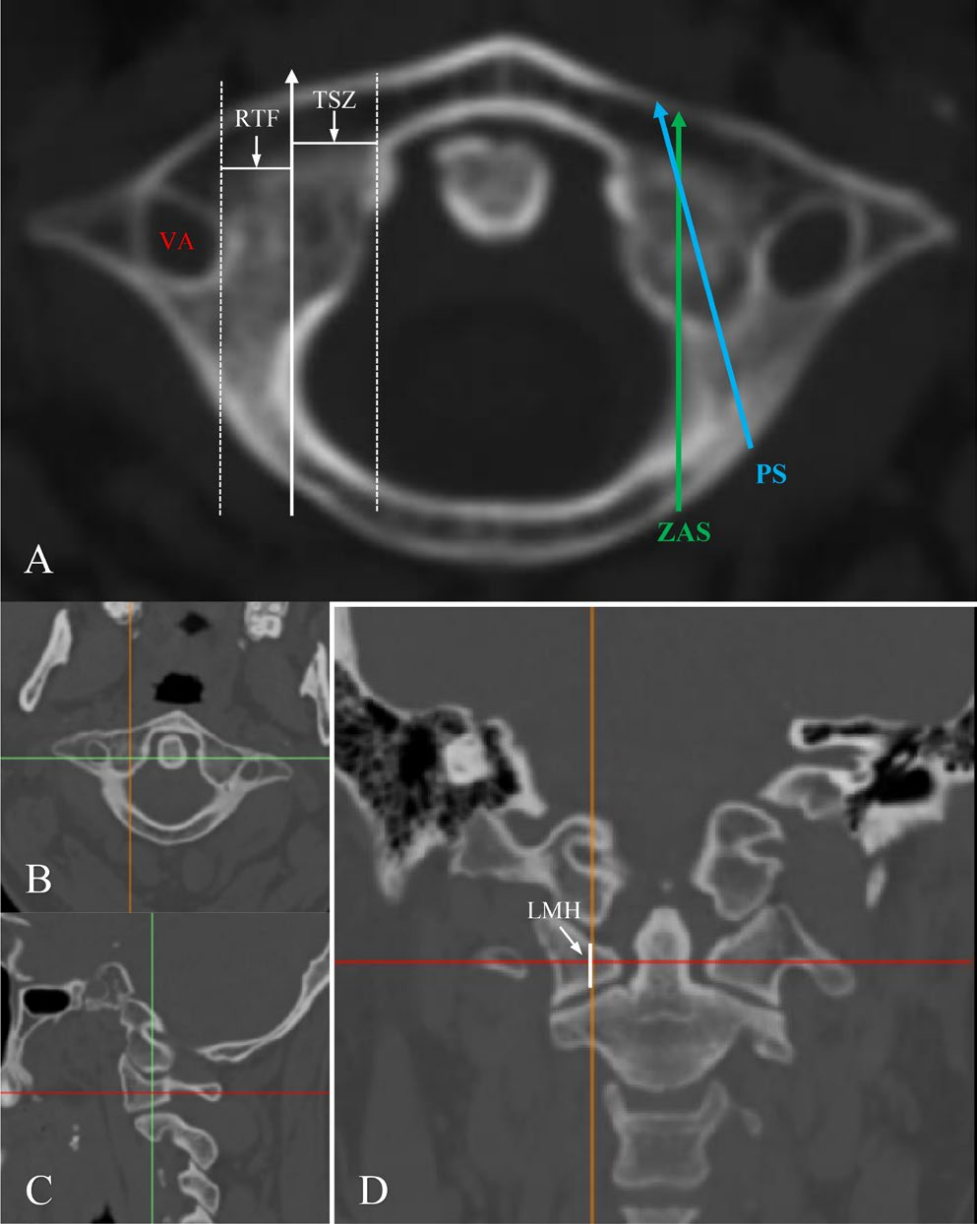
The axial, sagittal and coronal planes of the atlas in CT scans. (A) The white solid line with arrow was the ideal trajectory of C1ZAS. The white‐dashed lines were parallel lines of the trajectory of C1ZAS. RTF was the distance between the recess and the TF. TSZ was the distance between the recess and the inner of lateral mass that could form tricortical fixation. The blue solid line and green solid line represented the screw length of conventional PS and C1ZAS. (B‐D) LMH was measured at the coronal plane (white solid line) along the orientation of the C1ZAS trajectory at the axial and sagittal planes.
